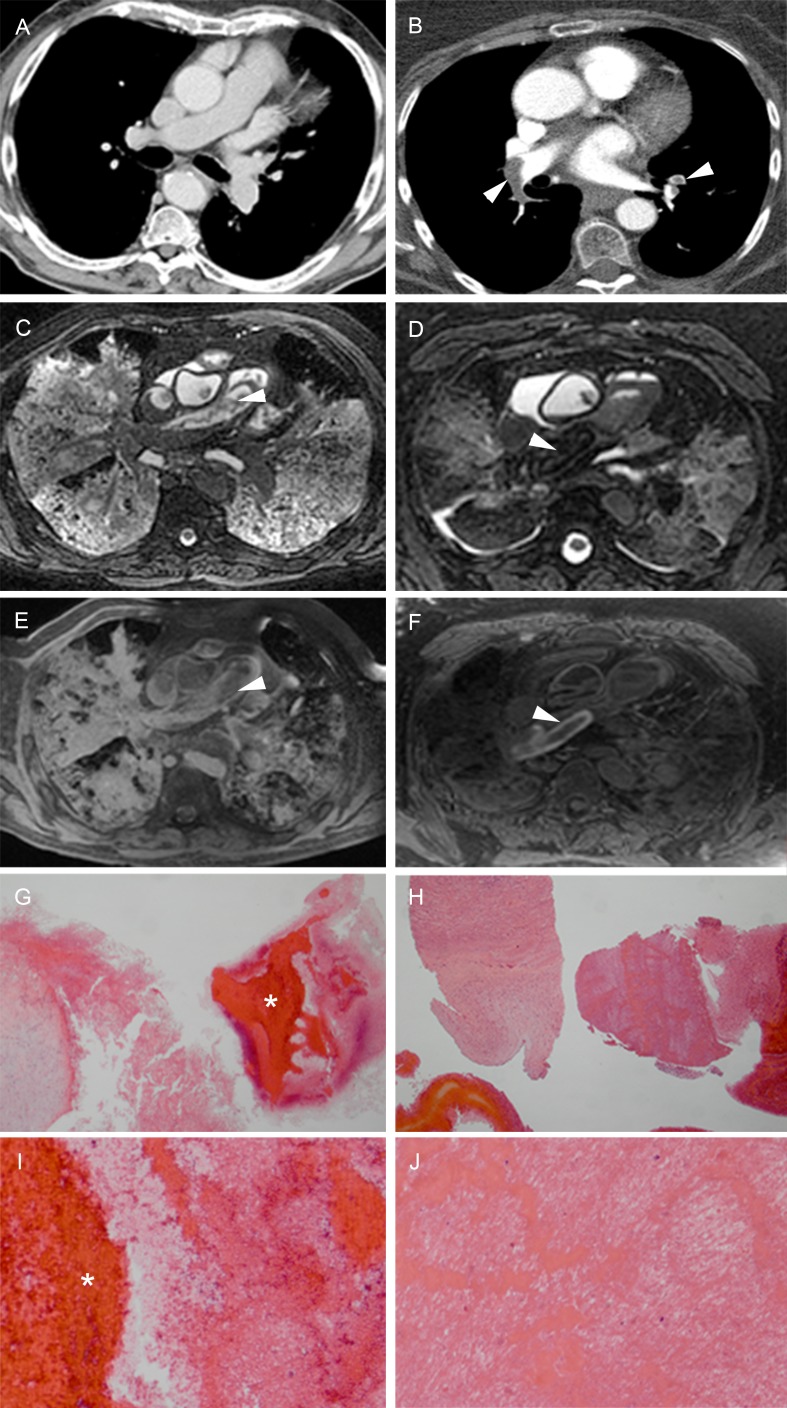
Pulmonary thrombo-embolism versus postmortem blood clot. (A/C/E) Antemortem CT (A) and T2w (C) and T1w (E) PMMR in the same patient. Antemortem CT (A) shows no abnormalities in the pulmonary arteries. PMMR (C/E) shows an irregularly shaped clot in the right pulmonary artery (C: arrowhead), with low T1 signal (E: arrowhead). (B/D/F) Antemortem CTA (B) and T2w (D) and T1w (F) PMMR in a patient with a thrombo-embolus. Antemortem CTA (B) shows a thrombo-embolus in the right and left pulmonary artery (arrowheads). PMMR (D/F) shows a thrombo-embolus in the right pulmonary artery (arrowheads). (G) HE, x16 original magnification, corresponding to image A/C/E. Wall of right pulmonary artery with a postmortem blood clot (asterisk). (I) HE, x50 original magnification, corresponding to image A/C/E. Higher power of blood clot showing blood with loosely arranged depositions of fibrin. (H) HE, x16 original magnification, corresponding to image B/D/F. Wall of pulmonary artery and thrombus. (J) HE, x50 original magnification, corresponding to image B/D/F. Wall of pulmonary artery and thrombus with dense depositions of fibrin and platelets, alternating with degenerated erythrocytes and white blood cells, thus constituting so-called lines of Zahn.
